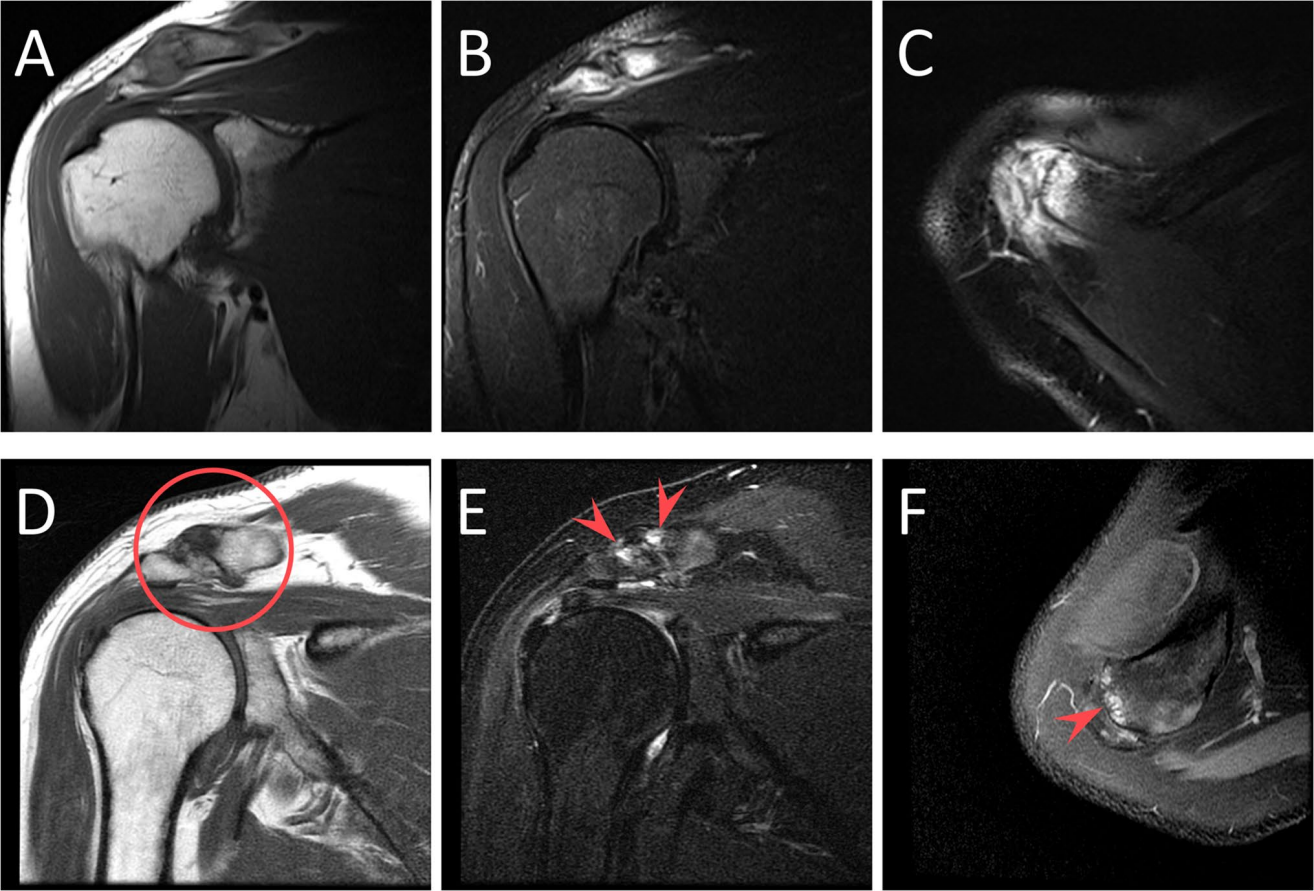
Coronal T1 (A), coronal STIR (B), and axial PD fat-suppressed (C) MR images demonstrating findings of post-traumatic distal clavicular osteolysis with involvement of the anterior acromion in a 46-year-old with shoulder pain for 2 months after a fall with hyperextension of the arm (A–C). Contrast this case with findings of severe osteoarthritis in a 65-year-old with chronic shoulder pain (D–F), in which coronal T1 (D), coronal STIR (E), and axial PD fat-suppressed (F) MR images demonstrate joint space narrowing with marginal osteophytes (circled, D) and subchondral cystic change without significant associated bone marrow edema (arrowheads, E and F)
